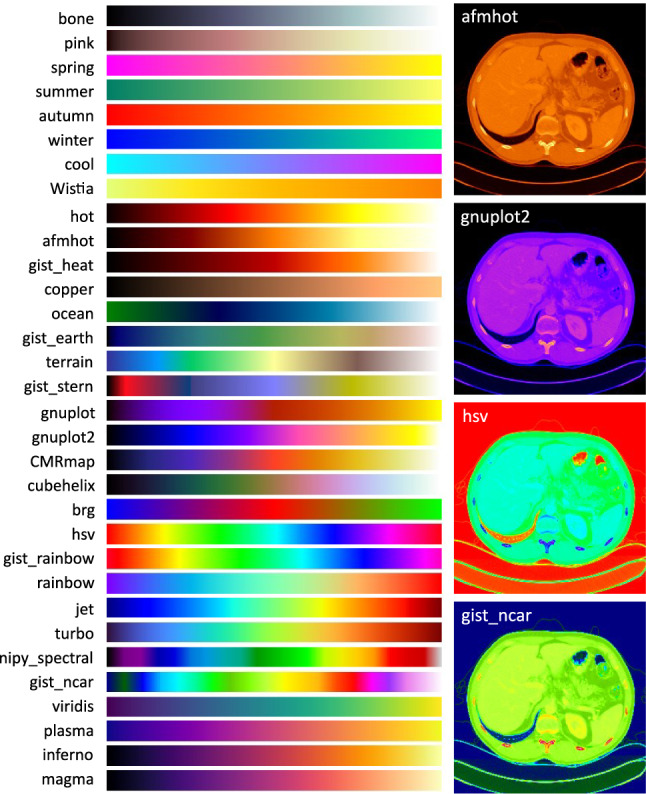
Selected colormaps and exemplary transformed CT slices
